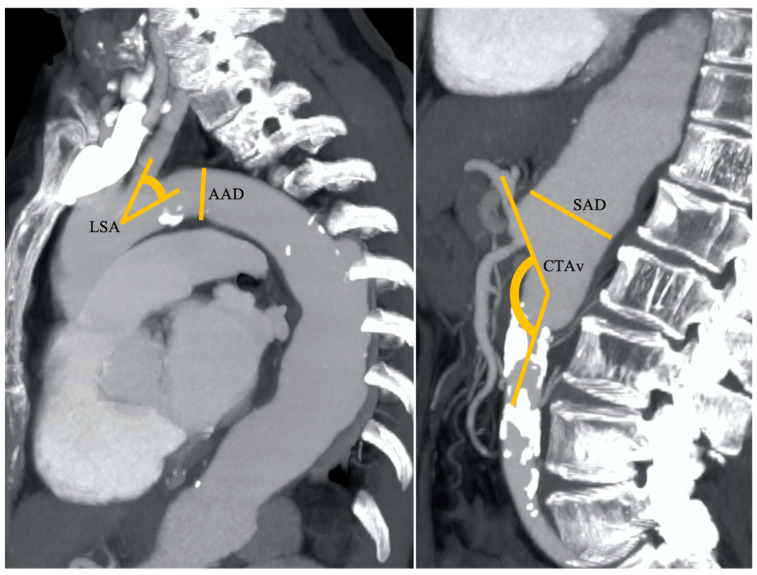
An 83-year-old patient with nodular HCC in the right liver lobe with a radial diameter (RD) of 2 mm (not shown) (0 points), left subclavian artery angle (LSA) of 45° (1 point), an aortic arch angulation (AAA) type III (1 point) with a diameter (AAD) of 32 mm (1 point), suprarenal aorta diameter (SAD) of 40 mm (1 point), and celiac trunk angle (CTA) of 130° (1 point). Final RAD-access score: 5, transfemoral access preferred for values between 3 and 6.
